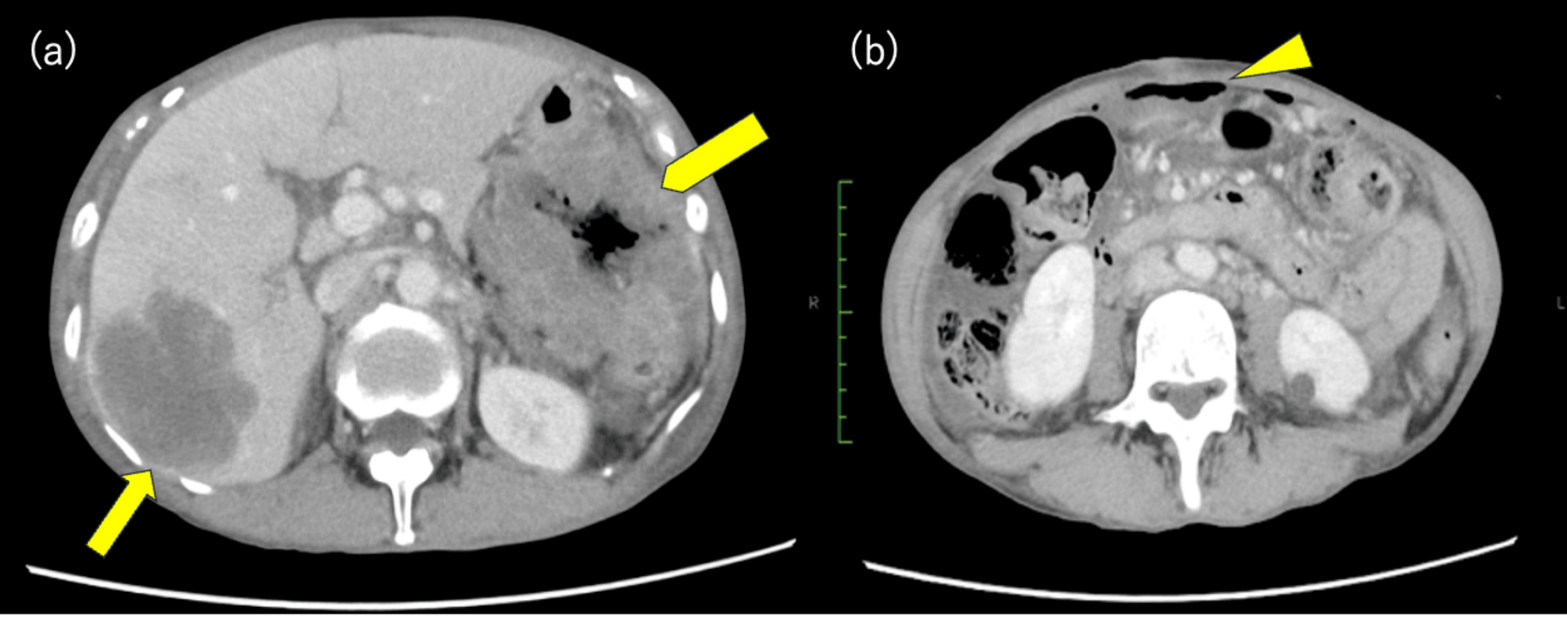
Abdominal computed tomography (CT) findings at presentation. (a) Multiple hepatic metastases (arrow) and a primary colorectal tumor with significant wall thickening (arrowhead); (b) free air in the abdominal cavity, indicative of intestinal perforation (triangle marker).
